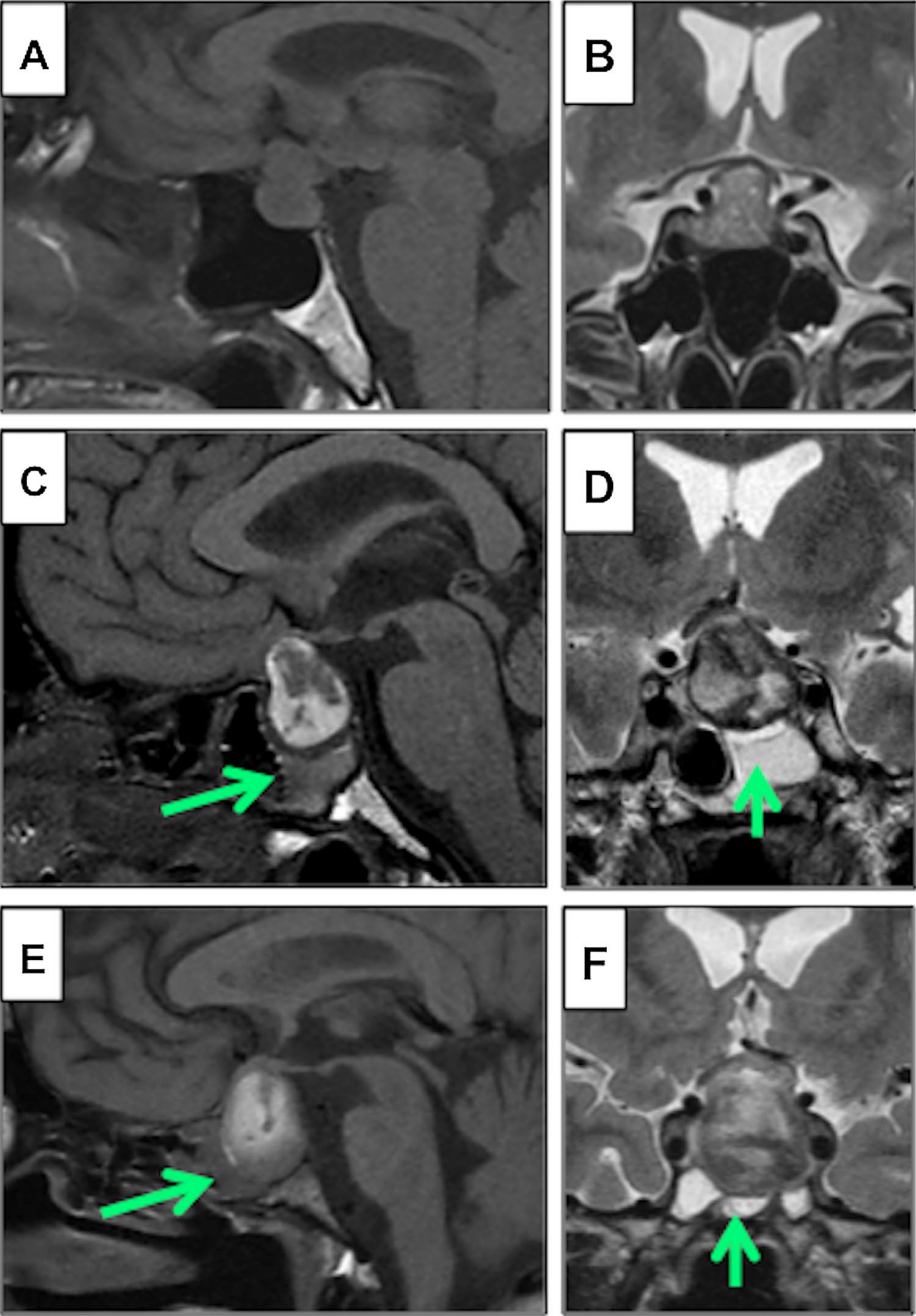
Examples of sphenoid sinus disease as measured by the sphenoid sinus mucosal thickness on sagittal T1-weighted (a, c, e) and coronal T2-weighted (b, d, f) MRI sequences. a, b A 66 year old male with an incidental NFPA with chiasmal compression, but no significant SSMT preoperatively. c, d A 56 year old male presented with acute onset headaches, nausea, bilateral field defects and reduced visual acuity. MRI showed increased signal on T1-weighted imaged consistent with haemorrhage/infarction of a pituitary adenoma and there was significant SSMT (2.5 mm) with obliteration of the left side (25–50%: *green arrow*). e, f A 24 year old male presented acutely with confusion and refractory hyponatraemia. Imaging revealed high signal on T1-weighted MRI and there was marked SSMT (5 mm), with near complete obliteration of the sinus space bilaterally (75–100%: *green arrow*)
